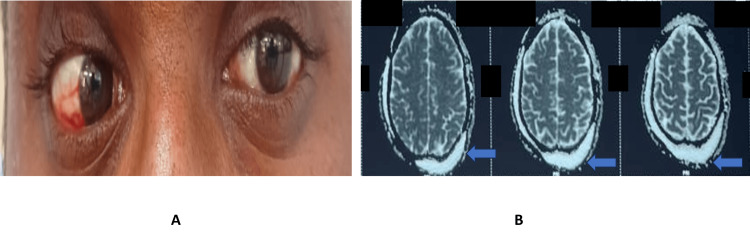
Clinical photograph and MRI of the brain demonstrating isolated LR palsy. (A) Right eye lateral rectus palsy. (B) MRI showing subarachnoid hemorrhages in sulci of frontoparietal lobes.
